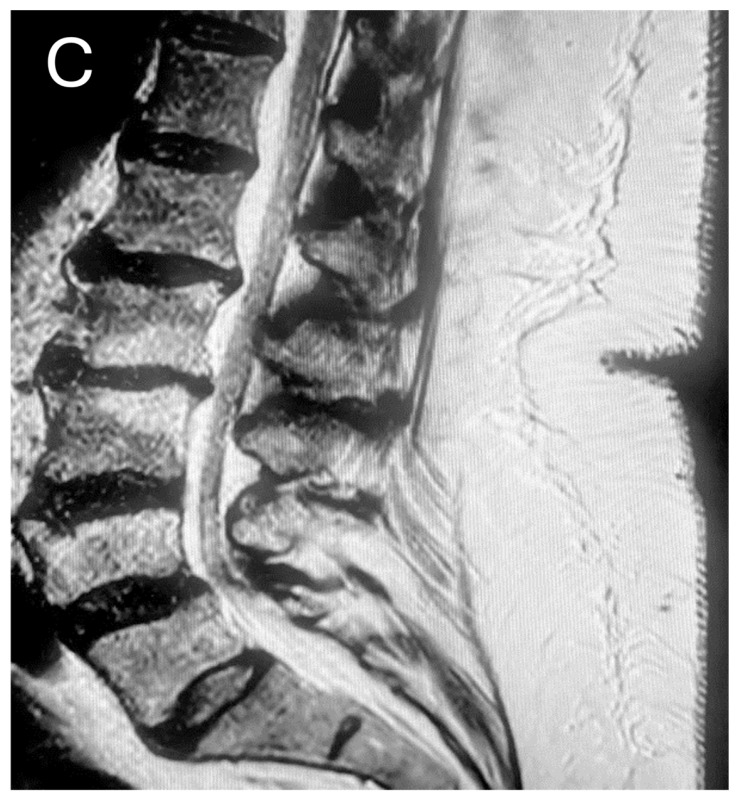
Three-dimensional slicer-based segmentation (A), 3D-printed model (B), and corresponding sagittal lumbar T2-weighted MRI scan (C). The patient suffered from an intraforaminal lumbar disc prolapse that was evident on more lateral slices, which are not presented here.
